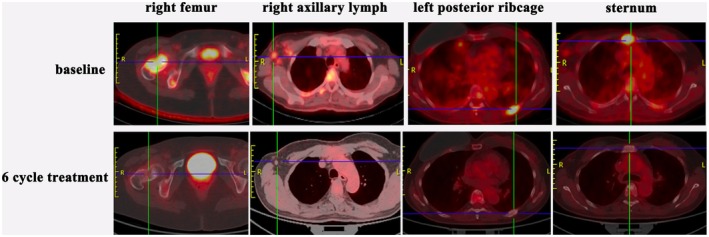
PET‐CT evaluation after first‐line THP treatment.
